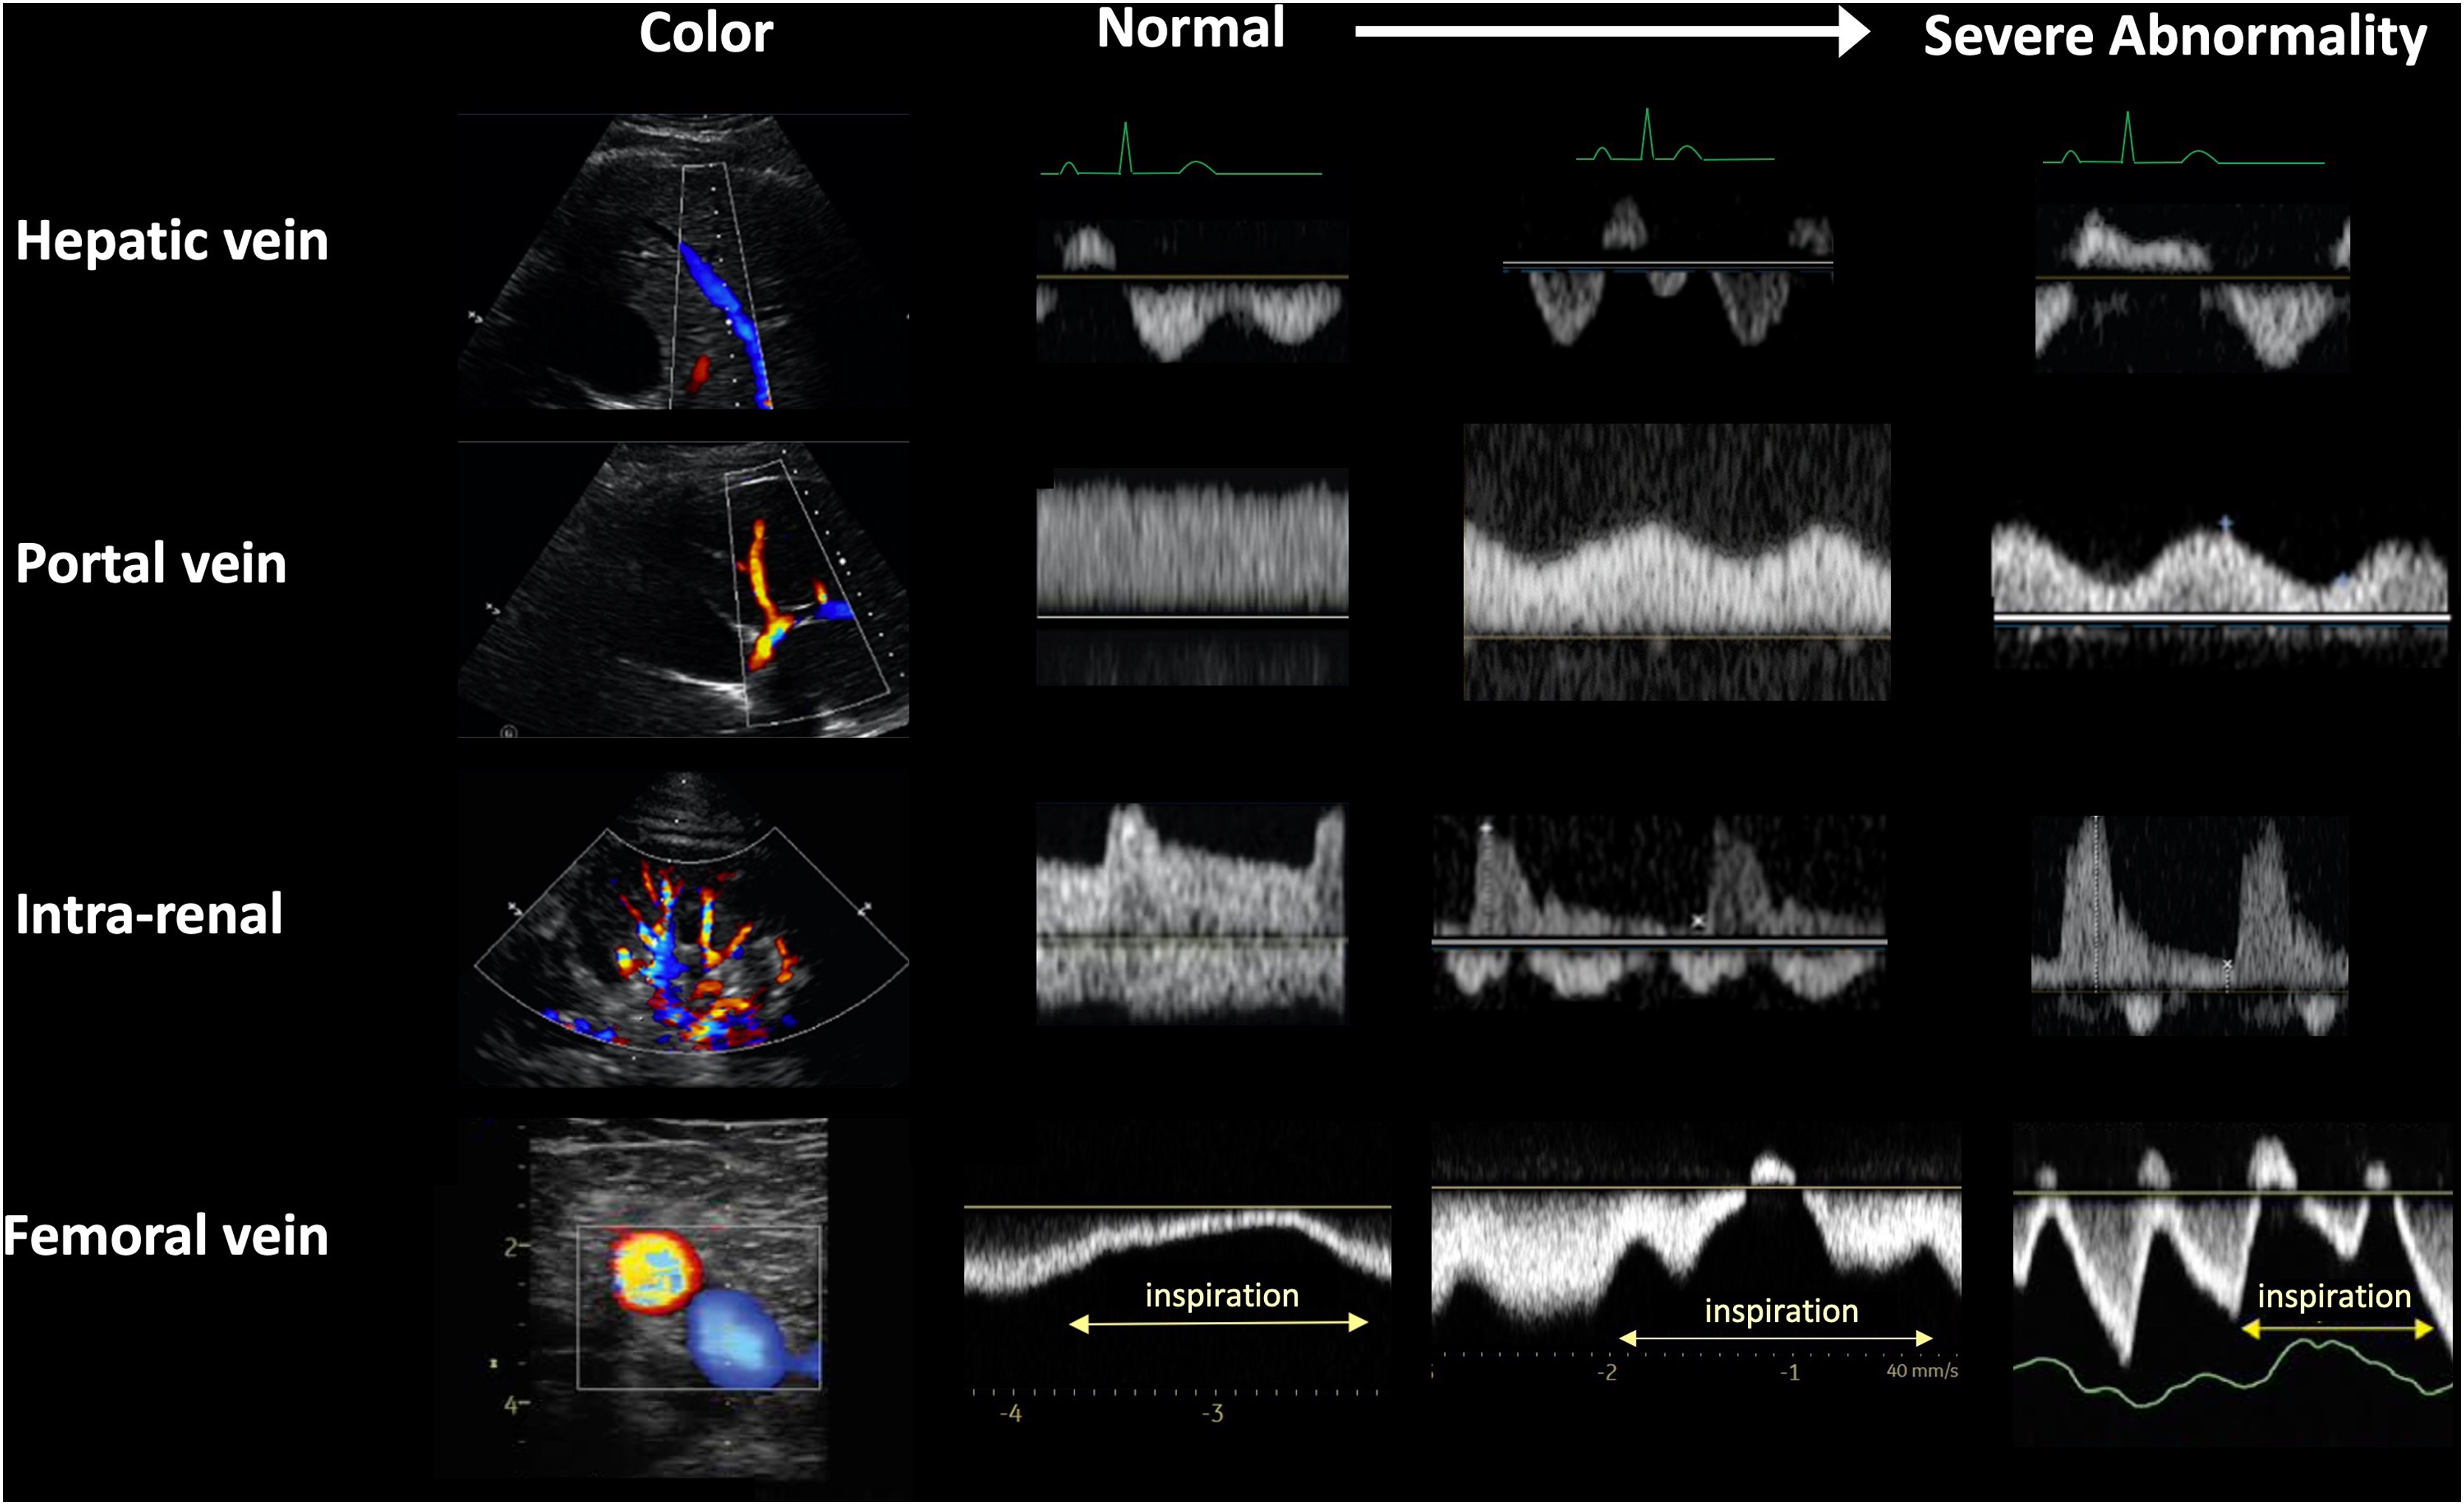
Doppler assessment of systemic veins with point-of-care ultrasound. Venous congestion causes abnormal waveform patterns.
